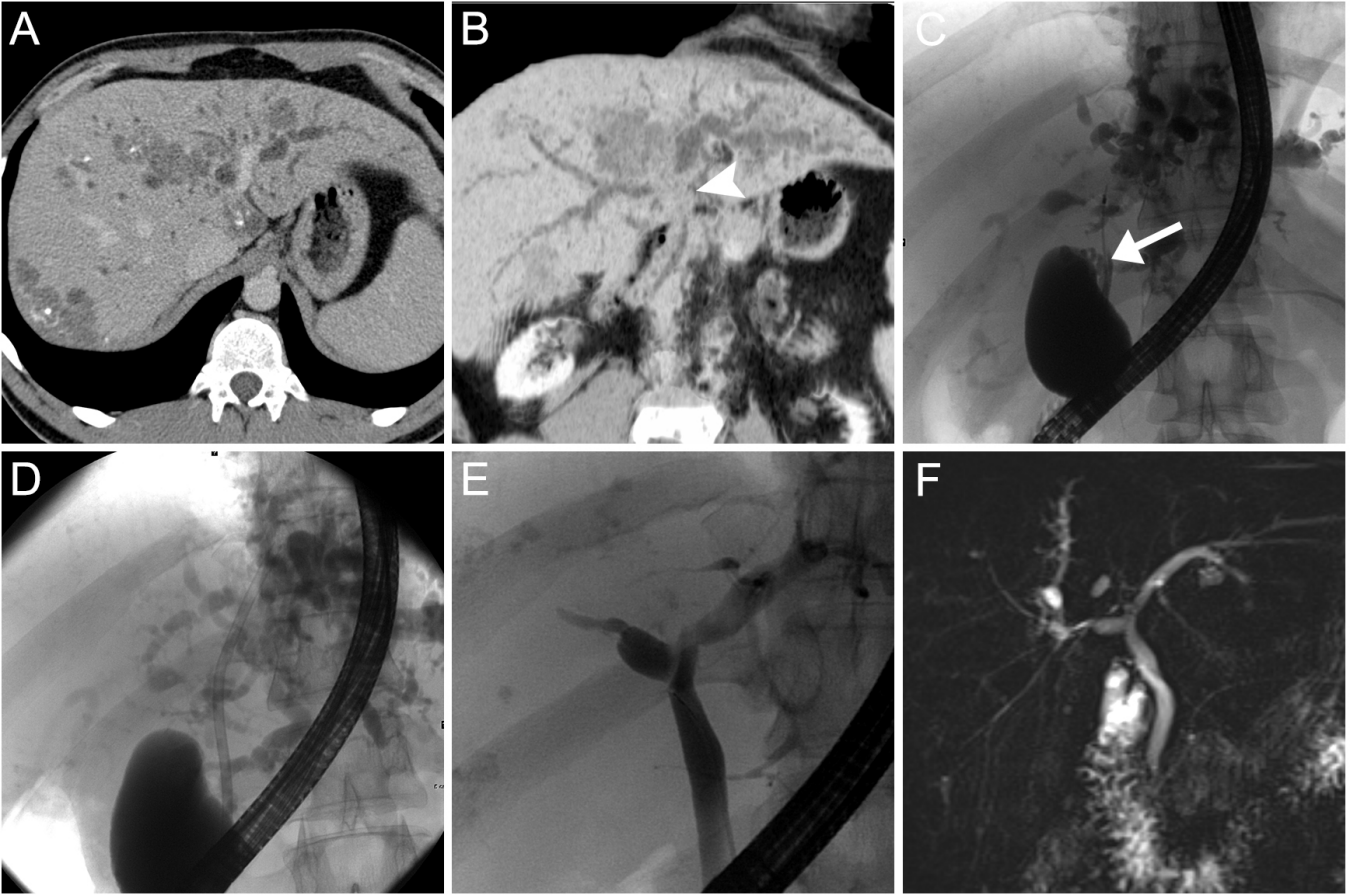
Imaging and ERC findings patient 1. CT scans in axial orientation show multifocal hypovascular calcified mass lesions and obstructive cholestasis with predominance on the left side (A). Minimum intensity projection in oblique coronary orientation visualizes the central occlusion of both bile ducts (B, arrow head). With ERC and initial placement of a plastic stent (which becomes occluded early) and subsequent serial balloon dilation (C, D) patency of bile ducts was regained (E). MRCP confirms sustained clinical success of bile duct recovering (F).
